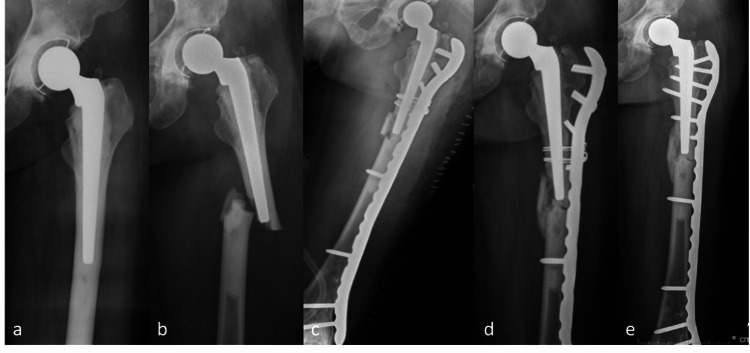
The radiographs from Case 2 a) post-operative total hip arthroplasty before APFF occurred, b) complete fracture originated from the cortical beak with medial spike and minimal comminution, c) immediate post-operative ORIF with plate and screws with wiring, bone graft, and demineralized bone matrix, d) proximal fixation failure with varus malalignment of the fracture at four months after fixation, and e) revision surgery with plate and screw.
